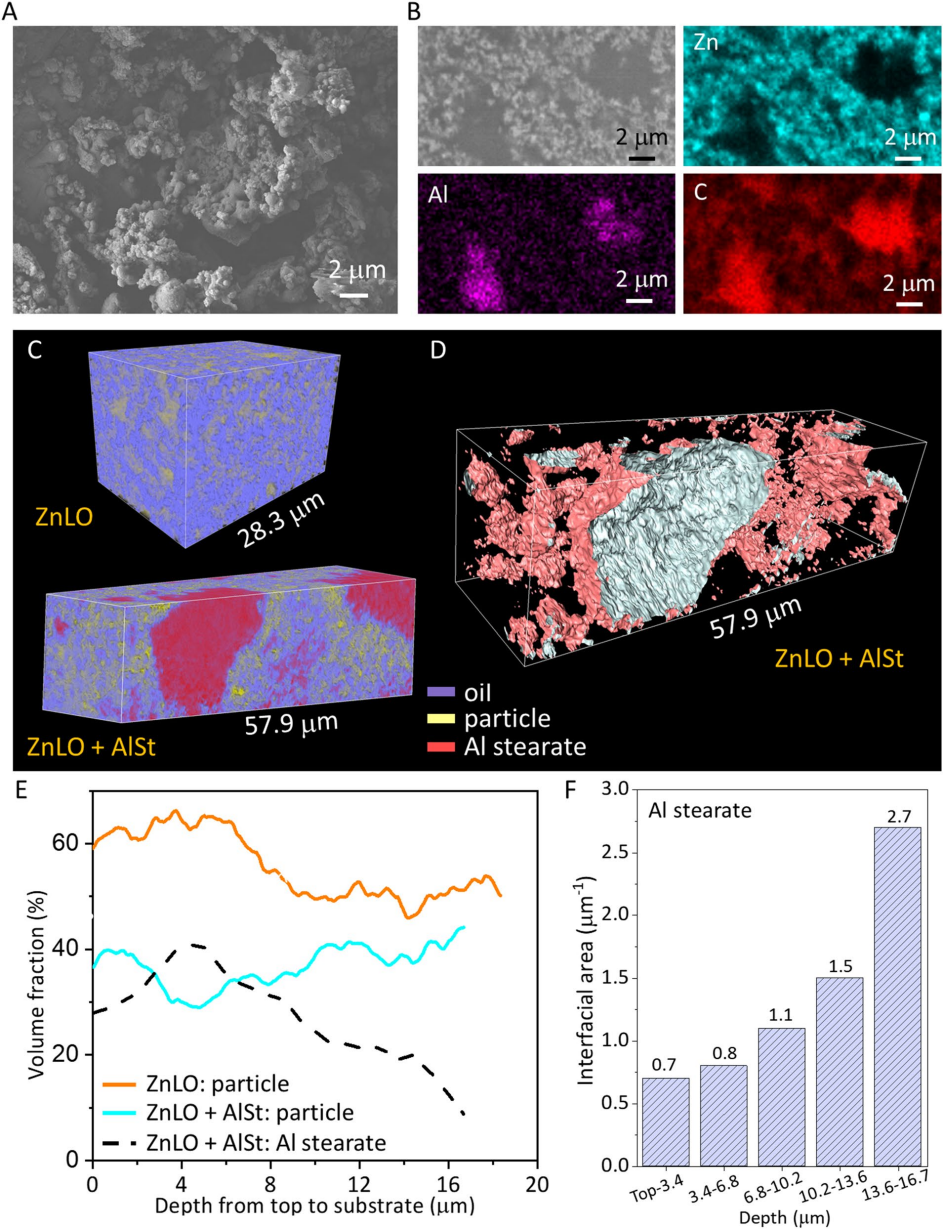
Characterization of the effect of Al stearate. (A) Surface morphology of Al stearate powder. (B) Cross-sectional image and EDS distribution maps for Zn, Al and C in the ZnLO + AlSt sample. (C) 3D visualization of ZnLO and ZnLO + AlSt samples by X-ray nano-tomography. (D) Surface structure and shape of Al stearate agglomeration. (E) Volume ratio of particles and Al stearate in the ZnLO and ZnLO + AlSt samples. (F) Interfacial area distribution of Al stearate agglomerates at different depths.
